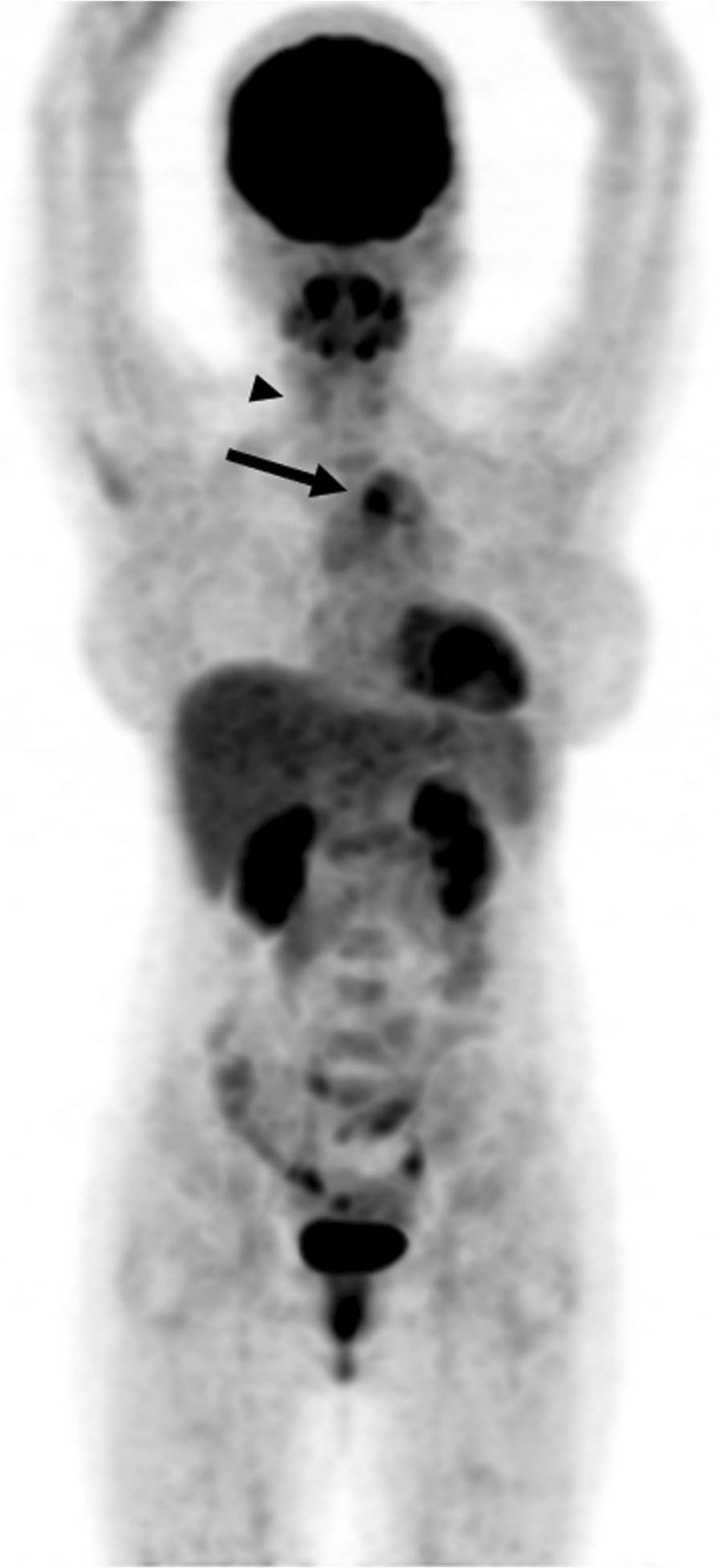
[F-18]FDG PET/CT scan of a 22-year-old woman diagnosed with Takayasu arteritis. [F-18]FDG uptake is present in the aortic arch (arrow). Note the diffuse [F-18]FDG uptake in thyroid glands, indicative of thyroiditis (arrowheads)
